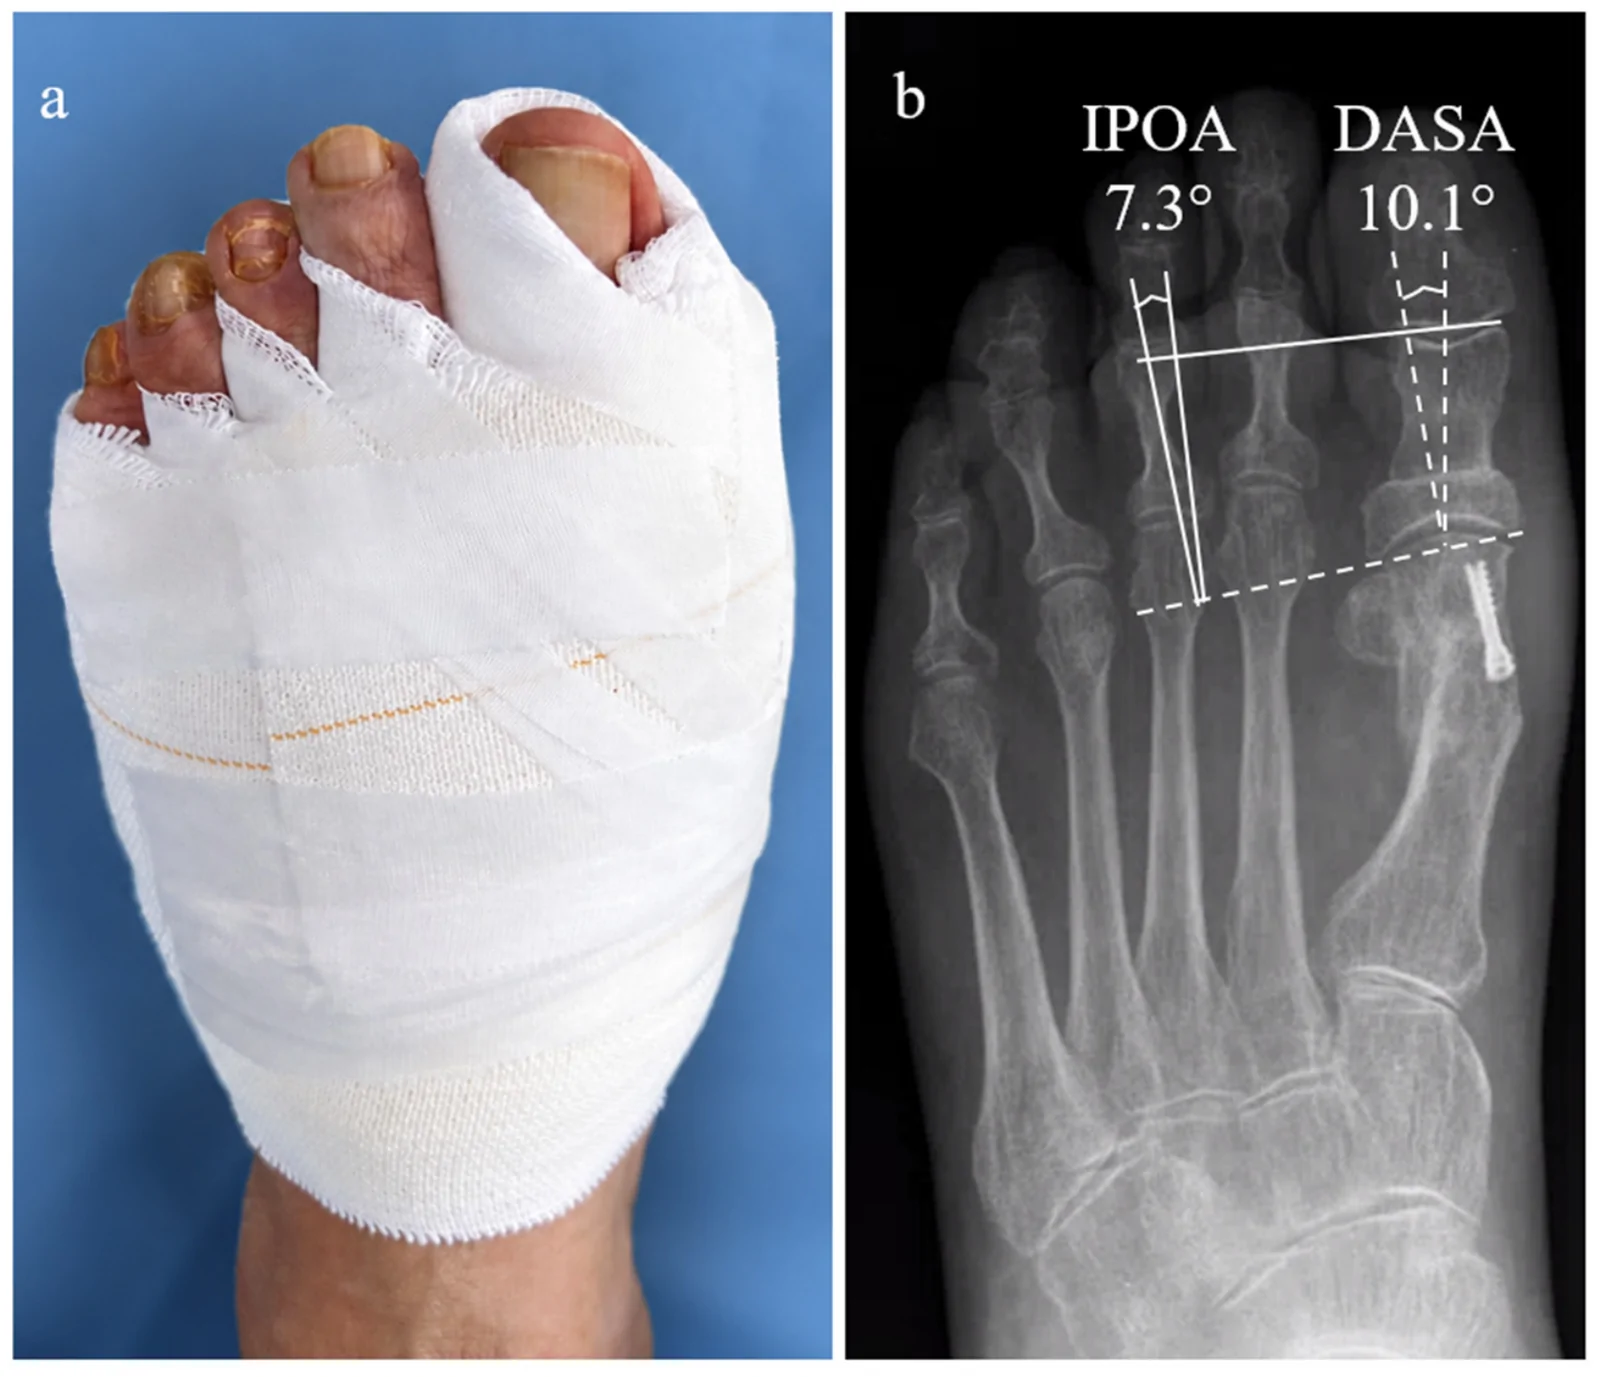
Postoperative bandage and X-ray. (a) Corrective postoperative bandage with varus thrust; the figure shows three gauze strips wrapped around the great toe and a single gauze strip around each of the lesser toes. The gauze strips are wrapped inward, creating a spacer effect between the toes while simultaneously applying a varus-directed corrective force. They are maintained in position using a semi-rigid double-sided adhesive bandage, and additional varus-directed adhesive strips are applied to complete the dressing. (b) Distal articular set angle (DASA—dashed lines) and interphalangeal joint obliquity angle (IPOA—solid lines) both measured on postoperative X-ray.
